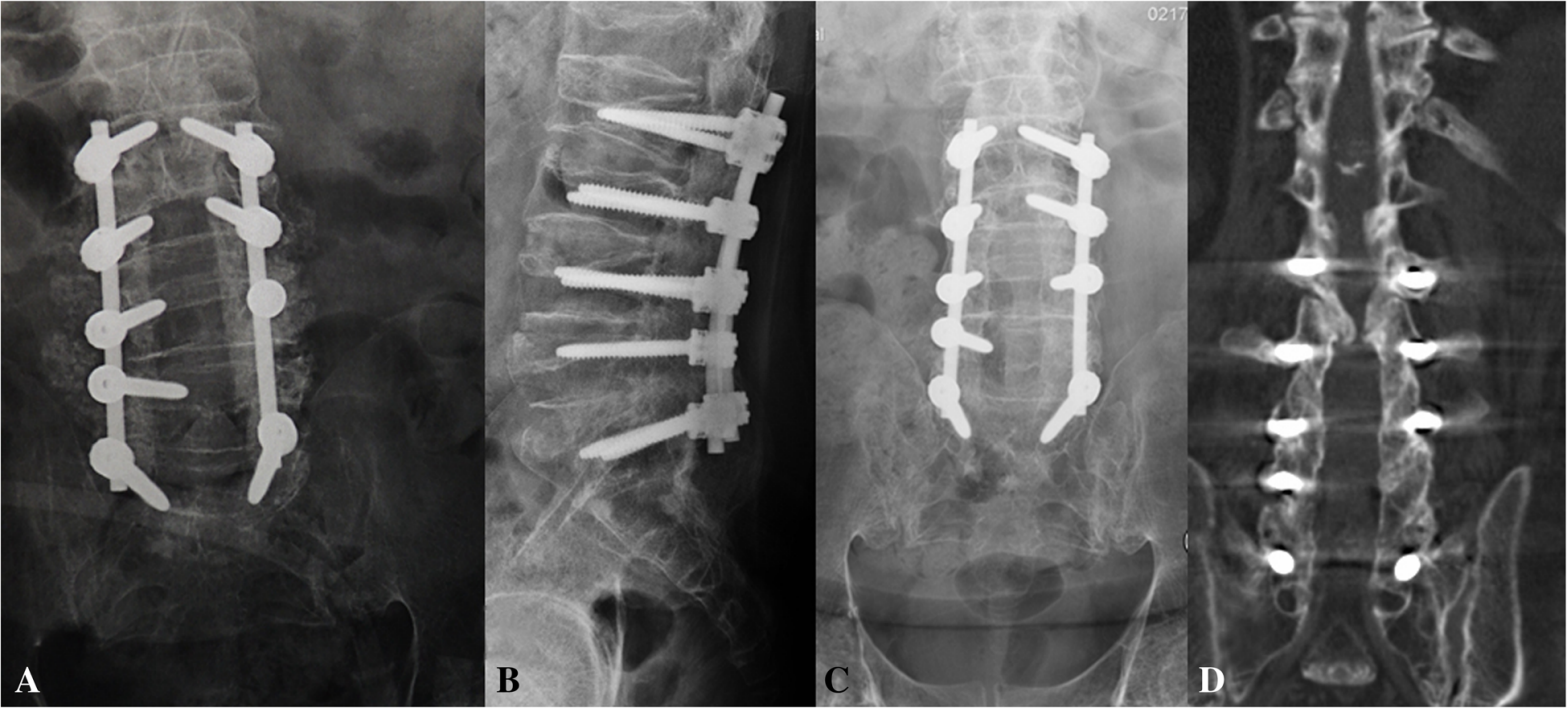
Extensive decompression of lumbar canal with internal fixation from L2 to S1 was implemented during the first surgery (a). X-ray and CT images suggest facet joint fusion 5 years later (b–d)
